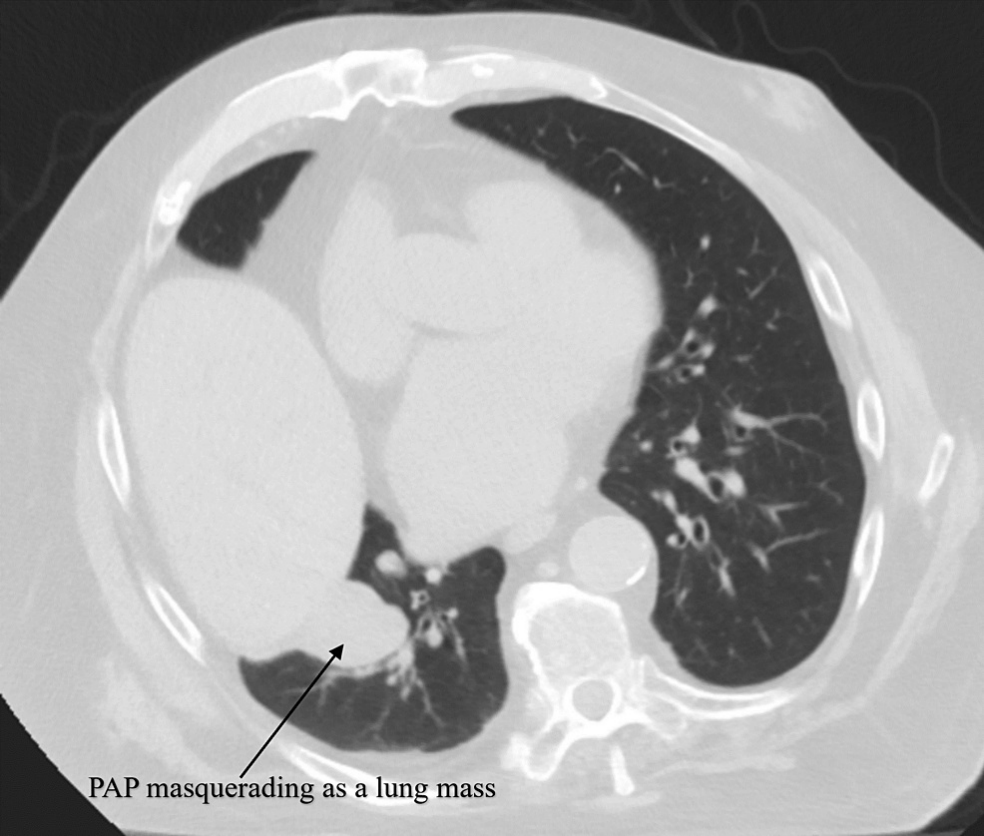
Noncontrast CT scan of lungs five years ago at the time of initial presentation PAP, pulmonary artery pseudoaneurysm
